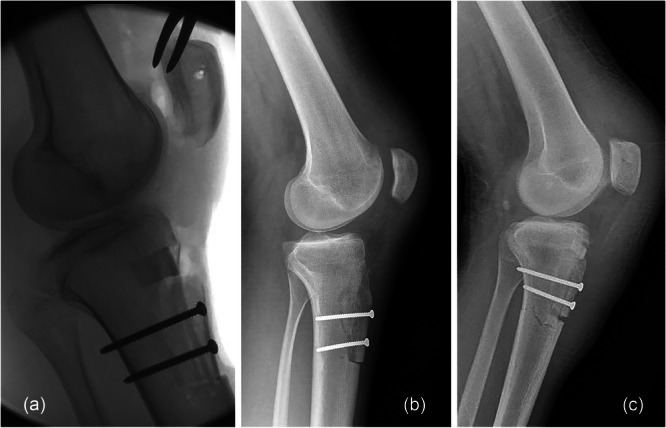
Sagittal tibial image. (a) Interoperative fluoroscopic image. (b) Sagittal radiograph: 18 days postop. (c) Sagittal radiograph 28 days postop with fracture line clearly evident.
